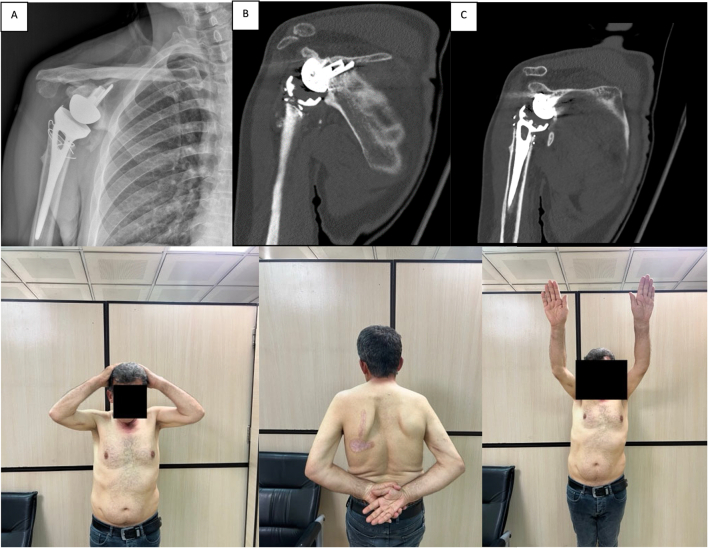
At one-year follow-up, radiographic and CT evaluations revealed no evidence of dislocation, component loosening, or any other abnormalities. Clinical photographs demonstrate that the patient has regained near-complete range of motion.
